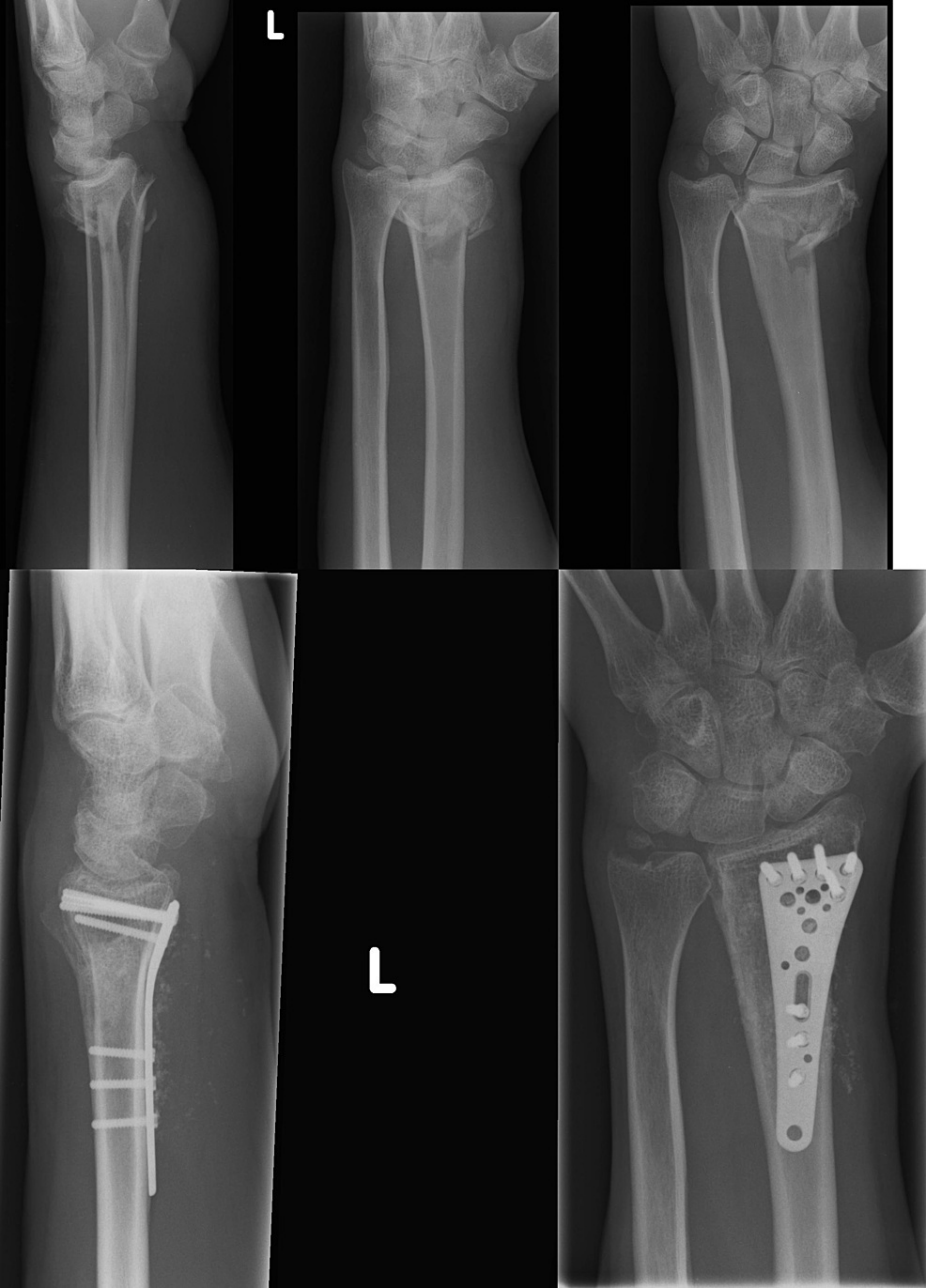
Pre and postoperative radiographs of an Aptus® trauma plate with a good raft of screws placed under subchondral bone and the AO 23-B1 fracture well reduced AO: Arbeitsgemeinschaft für Osteosynthesefragen
